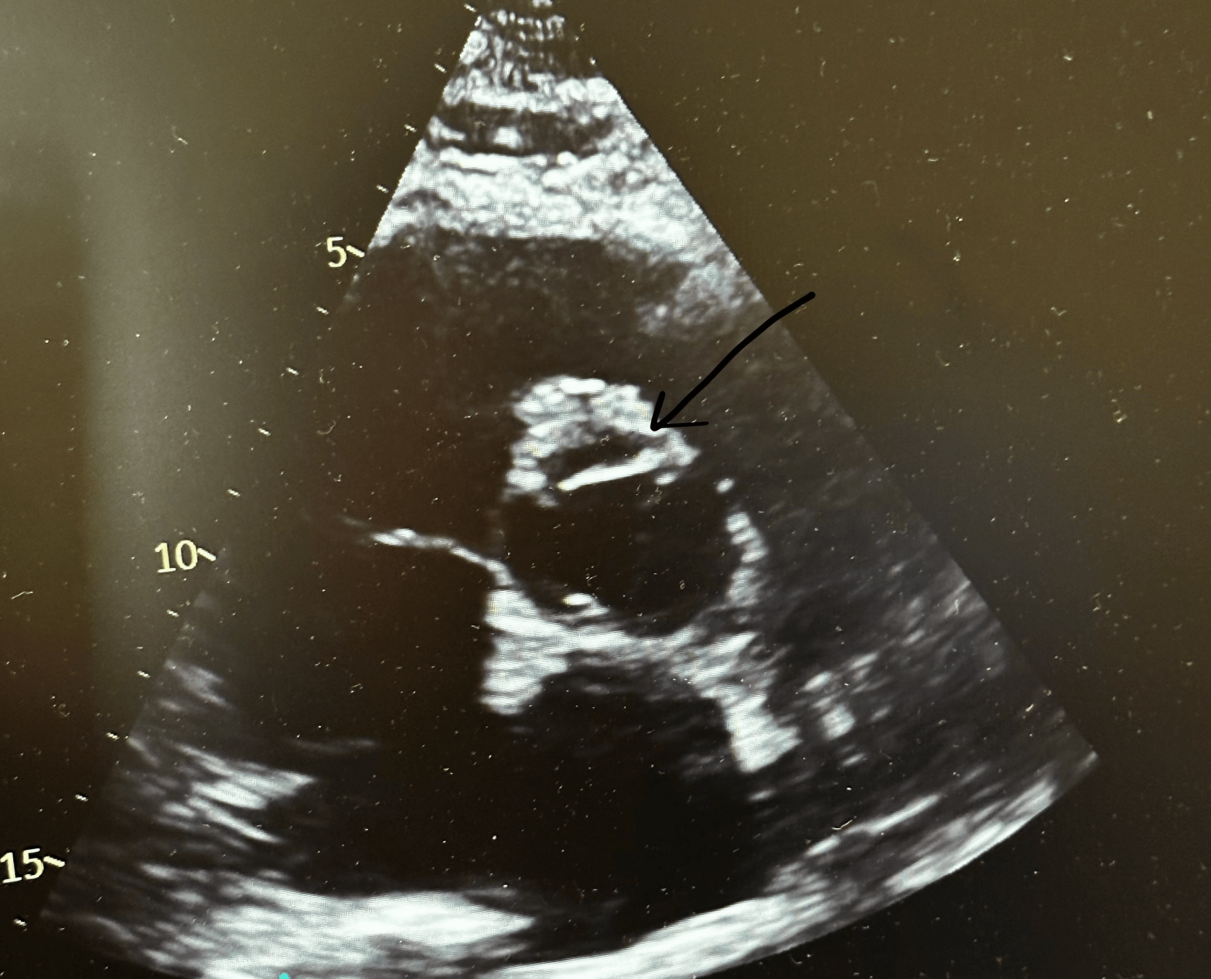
Transthoracic echocardiogram showing the dissecting flap in the aortic root (black arrow) in keeping with Stanford A dissection.
